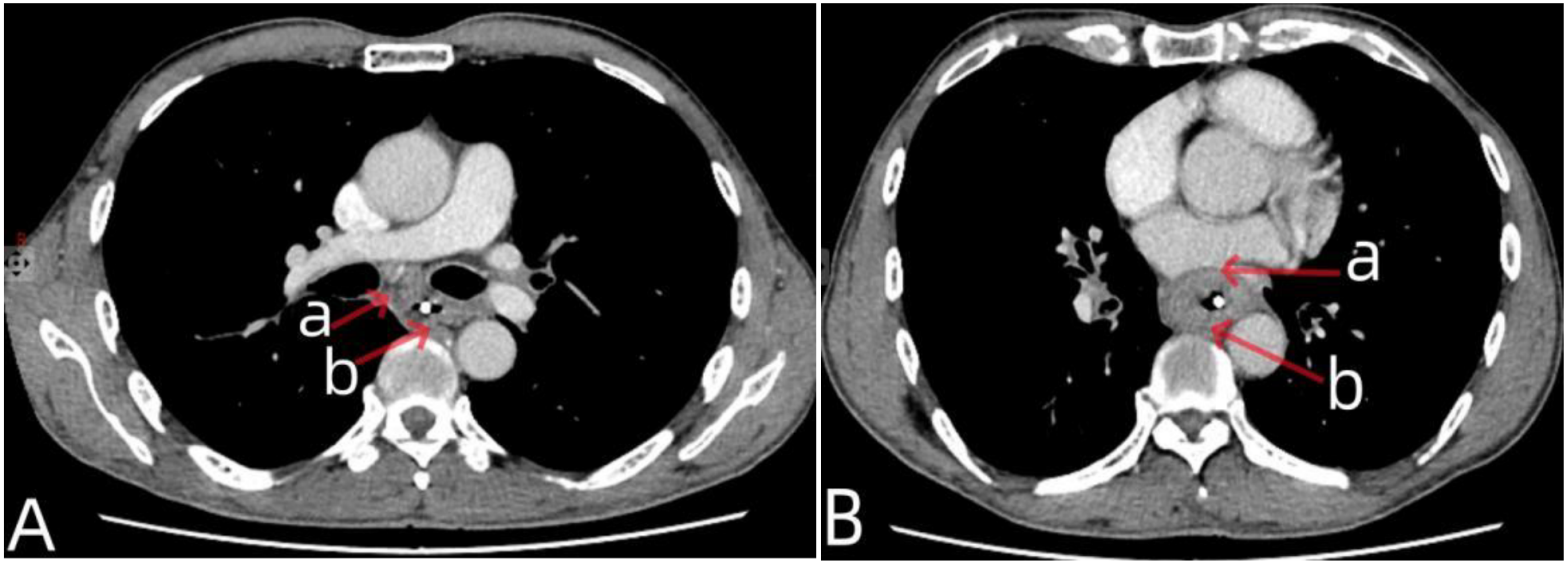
Chest CT data of the patient after neoadjuvant chemotherapy. In (A) the arrow a indicated that the enlarged subcarinal lymph nodes had shrunk after chemotherapy, the arrow b represented the esophageal tumor that had shrunk after chemotherapy. In (B) the arrow a indicated that there was a clear boundary between the esophageal tumor and pericardium, the arrow b indicated that there was a boundary between the esophageal tumor and the aorta.
